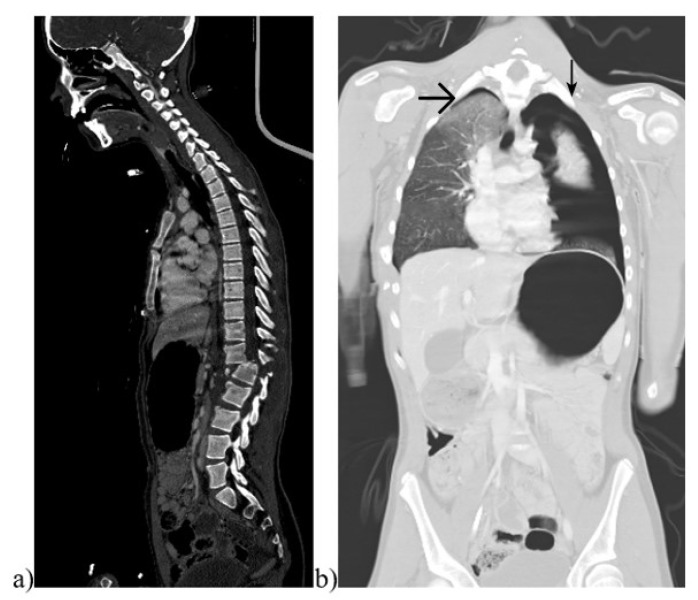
Images of a pediatric whole-body computed tomography (WBCT). Example of a 14-year-old girl with a traffic accident as a passenger in a car. CT images showing (a) a luxation fracture T12/L1 on sagittal plain in a bone window and (b) a pulmonary contusion and bilateral pneumothoraces on coronal plain in a lung window (marked by arrows).
